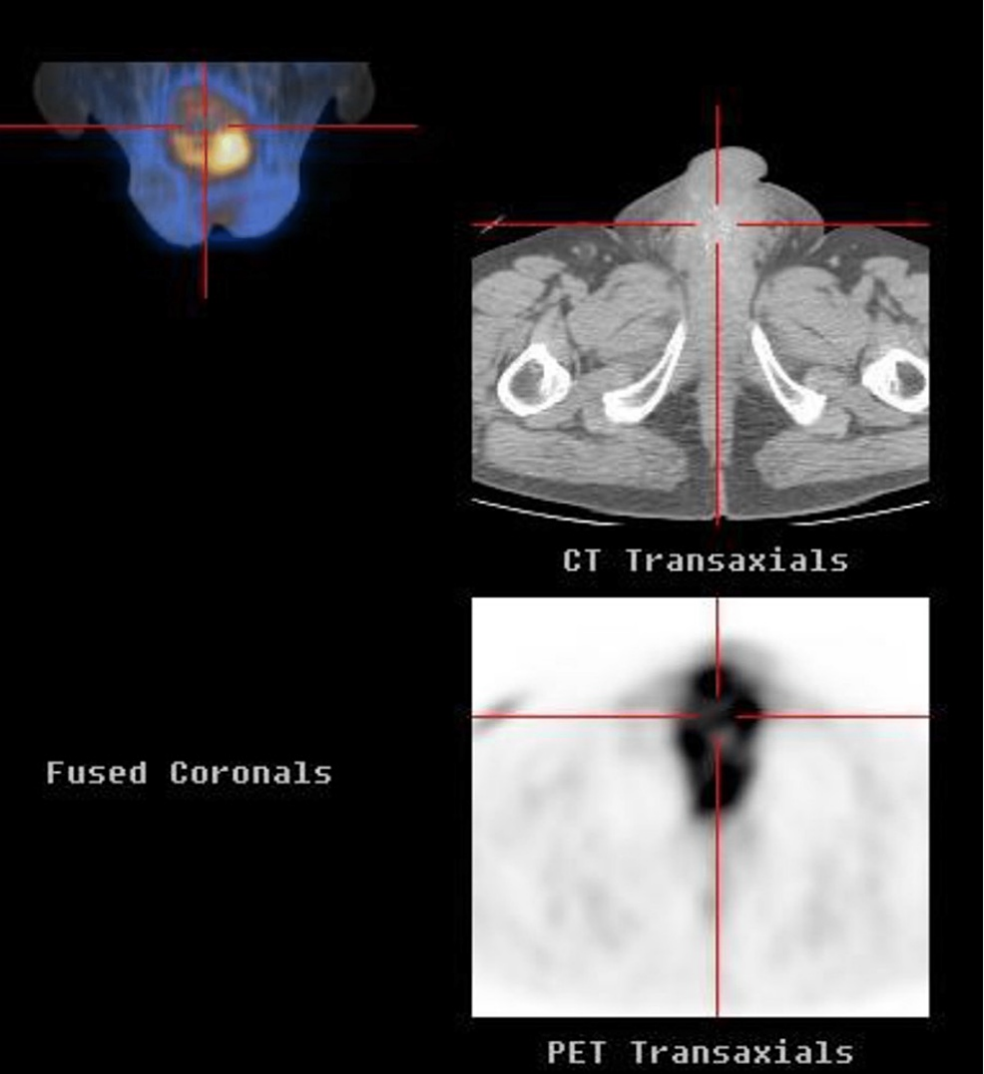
18F-FDG PET showing penile metastasis FDG PET: Fluorodeoxyglucose positron emission tomography
